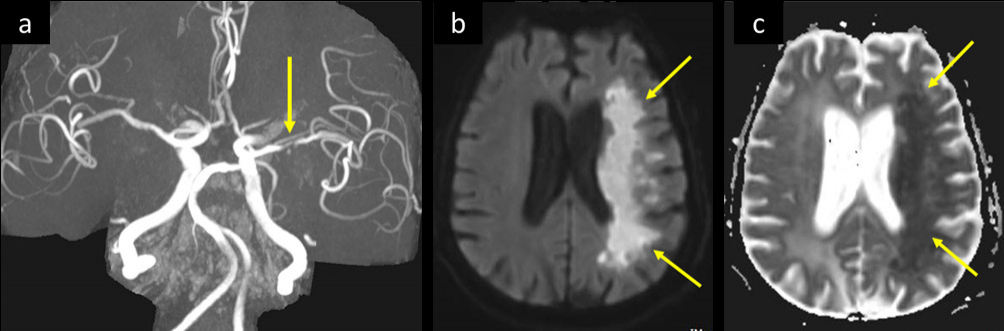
A 66-year-old male presented to ED with fatigue, cough, and fever, and was tested positive for COVID-19. He had multiple comorbidities including CAD, old cerebellar stroke, and DM. He developed pneumonia with rapid deterioration to ARDS which required intubation, and there was progressive deterioration of level of consciousness. (a) 3D-MIP- MRA shows severe stenosis of M1 segment of left MCA (arrow in a). (b) axial MRI DWI b1000 and corresponding (c) ADC map shows left-sided diffuse white matter diffusion restriction with bight signal intensity in (b) and low signal in (c), (arrows in b, c). ADC, apparent diffusion coefficient; CAD, coronary artery disease; DWI, diffusion-weighted image; ED,emergency department; DM, diabetes mellitus; MCA, middle cerebral artery; MIP, maximumintensity projection; MRA, MR angiography.
